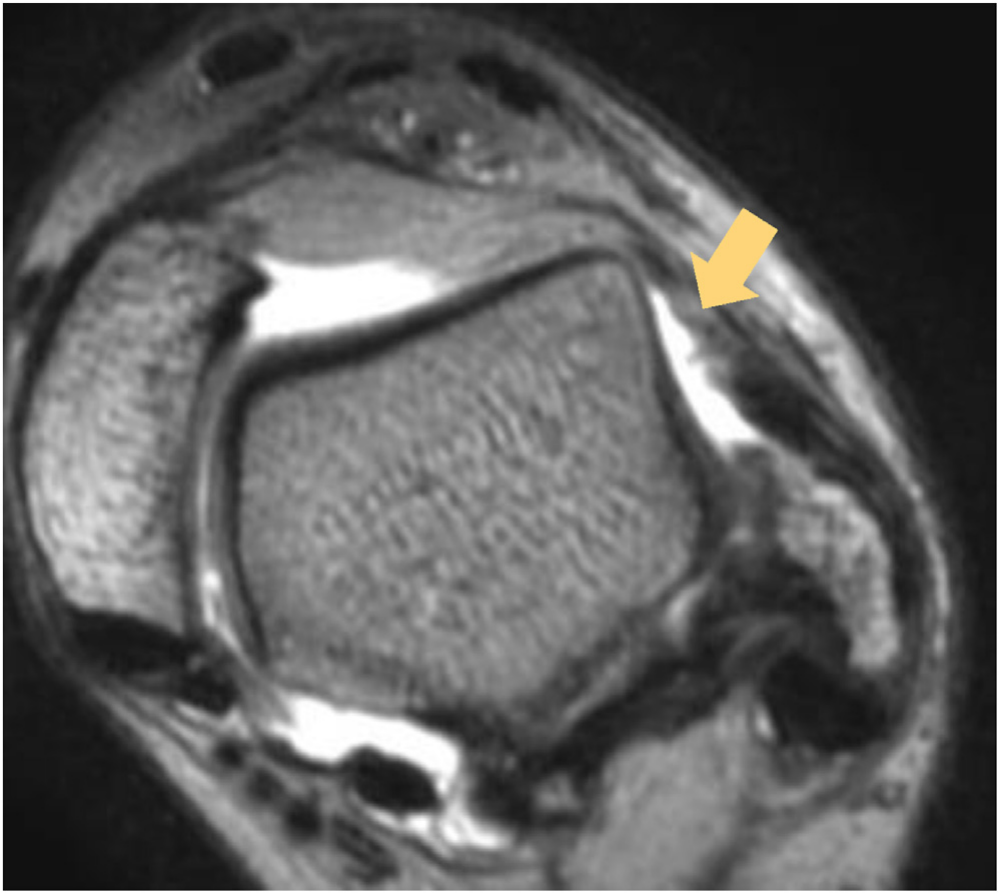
T2-weighted magnetic resonance imaging of anterior talofibular ligament (ATFL) injury site: oblique axial view. Normally, the fibers of the ATFL would appear as a bundle of low-intensity areas, but this image shows irregularity of the ATFL and detachment on the talar side (arrow).
